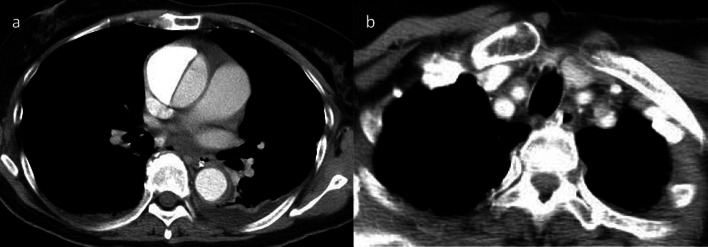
Chest CT shows double-barrel aortic dissection on the day of onset (a) and the dissected right common carotid artery with the false lumen thrombosed (b)
